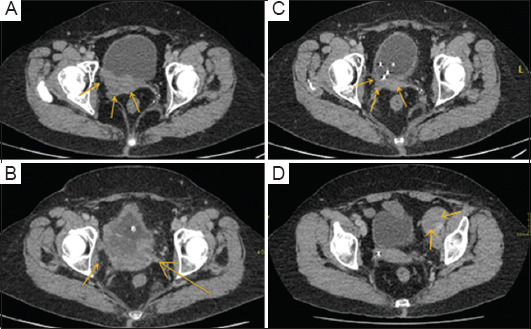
Computed tomography images taken before and after palliative radiotherapy to the bladder. (A) November 2019: Post-chemotherapy shows a large mass in the posterior bladder wall, (B) January 2020: Post-cycle two of atezolizumab shows increased size in posterior bladder wall mass, (C) May 2020: Post-radiotherapy and after 5 months of atezolizumab, the posterior bladder wall mass significantly shrank in size, (D) December 2021: End of atezolizumab scan after 2 years shows enlarged left external iliac node, estimated to be approximately 24–26 mm.
